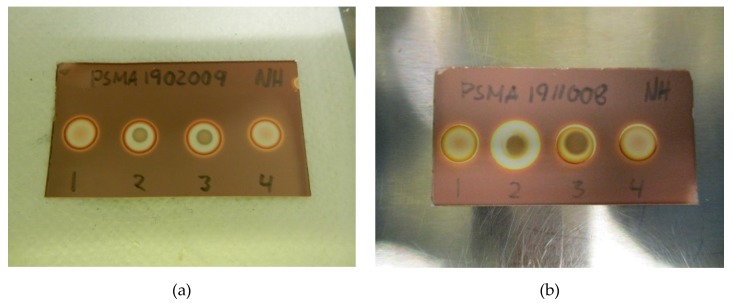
(a) Specificity without antioxidant: (1) [^18^F]PSMA-1007 + water, (2) TBA standard (0.26 mg/mL) + [^18^F]PSMA-1007, (3) TBA standard (0.26 mg/mL) + water, (4) matrix + water. (b) Specificity with antioxidant: (1) [^18^F]PSMA-1007 + H_2_O_2_, (2) TBA standard (0.26 mg/mL) + [^18^F]PSMA-1007 + H_2_O_2_, (3) TBA standard (0.26 mg/mL) + H_2_O_2_, (4) matrix + H_2_O_2_.
